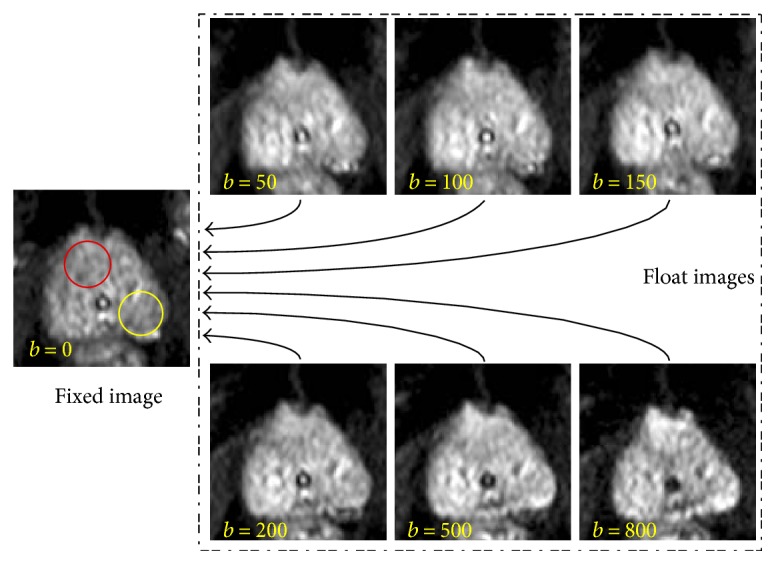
DWI sample acquired with *b* = 0, 50, 100, 150, 200, 500, and 800 in a 61-year-old patient. There was motion and deformation in the region of the prostate. For each patient, two types of elliptic ROIs are manually delineated on *b* = 0 s/mm^2^. The ROI A (red circle) includes the nidus of the prostate, and the ROI B (yellow circle) covers the normal region. The ROIs are propagated differently in the “no processing,” “affine,” and “FFD” schemes.
